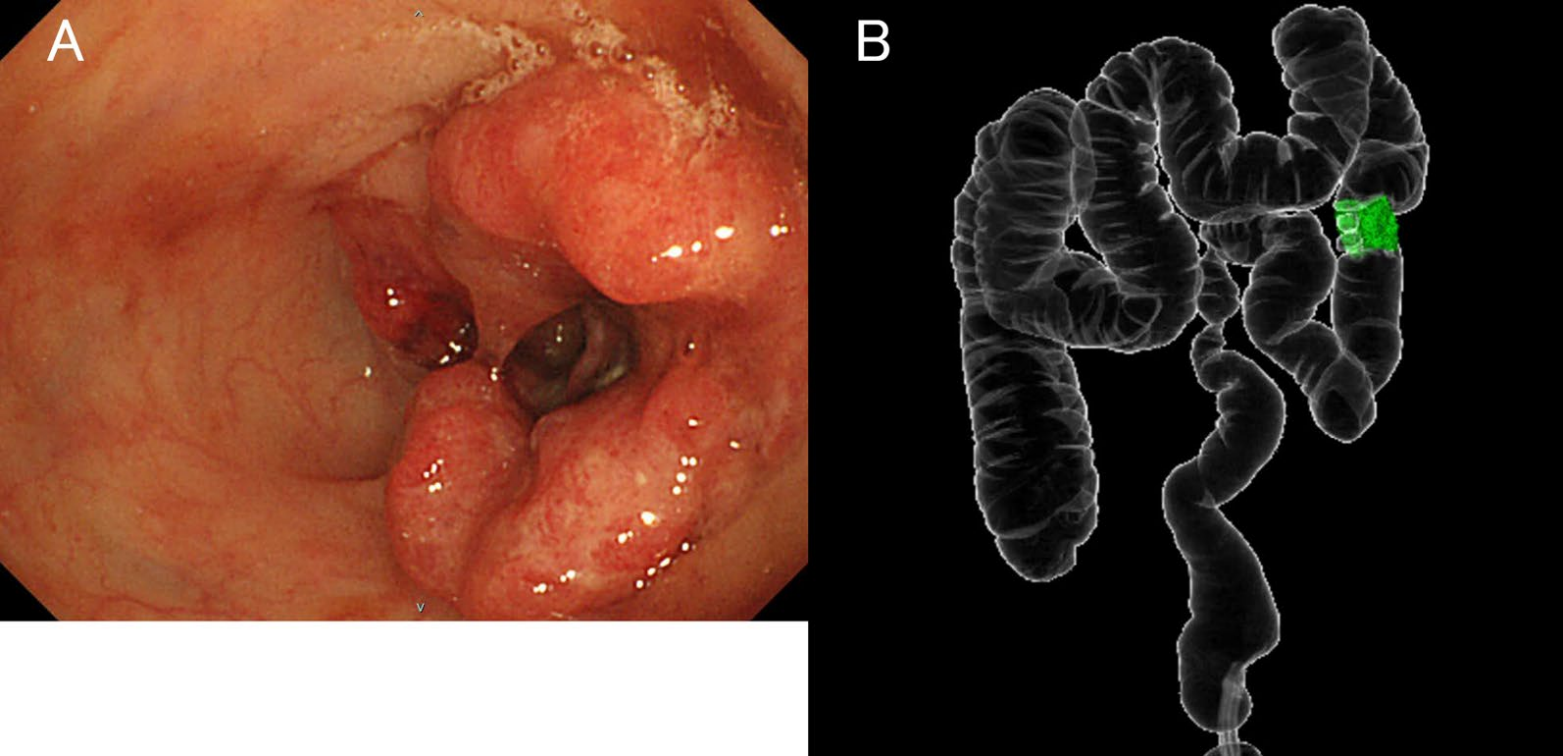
Colonoscopy and CT–colonography findings. A Colonoscopy revealed a semicircular type 2 lesion in the middle segment of the descending colon. B CT colonography showed a lesion in the middle segment of the descending colon
